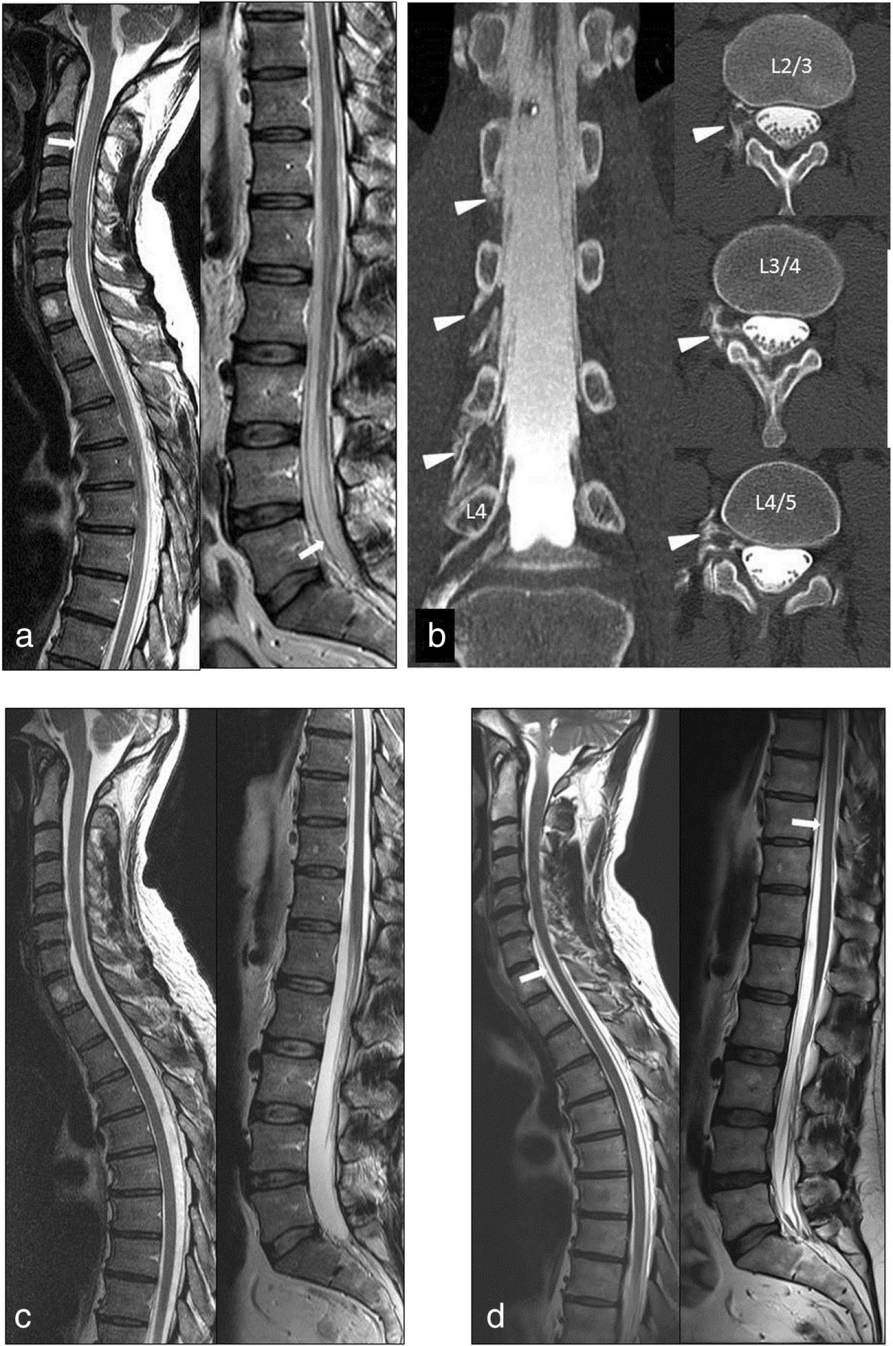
Initial sagittal T2-weighted MR images of the cervicothoracic and lumbar regions (a) demonstrate extensive anterior and posterior intraspinal fluid collections from C1 down to S1 (arrows). Coronal and axial reformatted CT myelography (b) reveals right-sided CSF leaks at the lumbar roots L2, L3, and L4 (arrowheads). Resolution of the intraspinal fluid collections is documented in sagittal T2-weighted MR images 1 month after a single lumbar CT-guided blood patch application (c). De novo formation of the intraspinal fluid collection (arrows) is observed without clinical symptoms in the late follow-up MR 3 years later (d)
